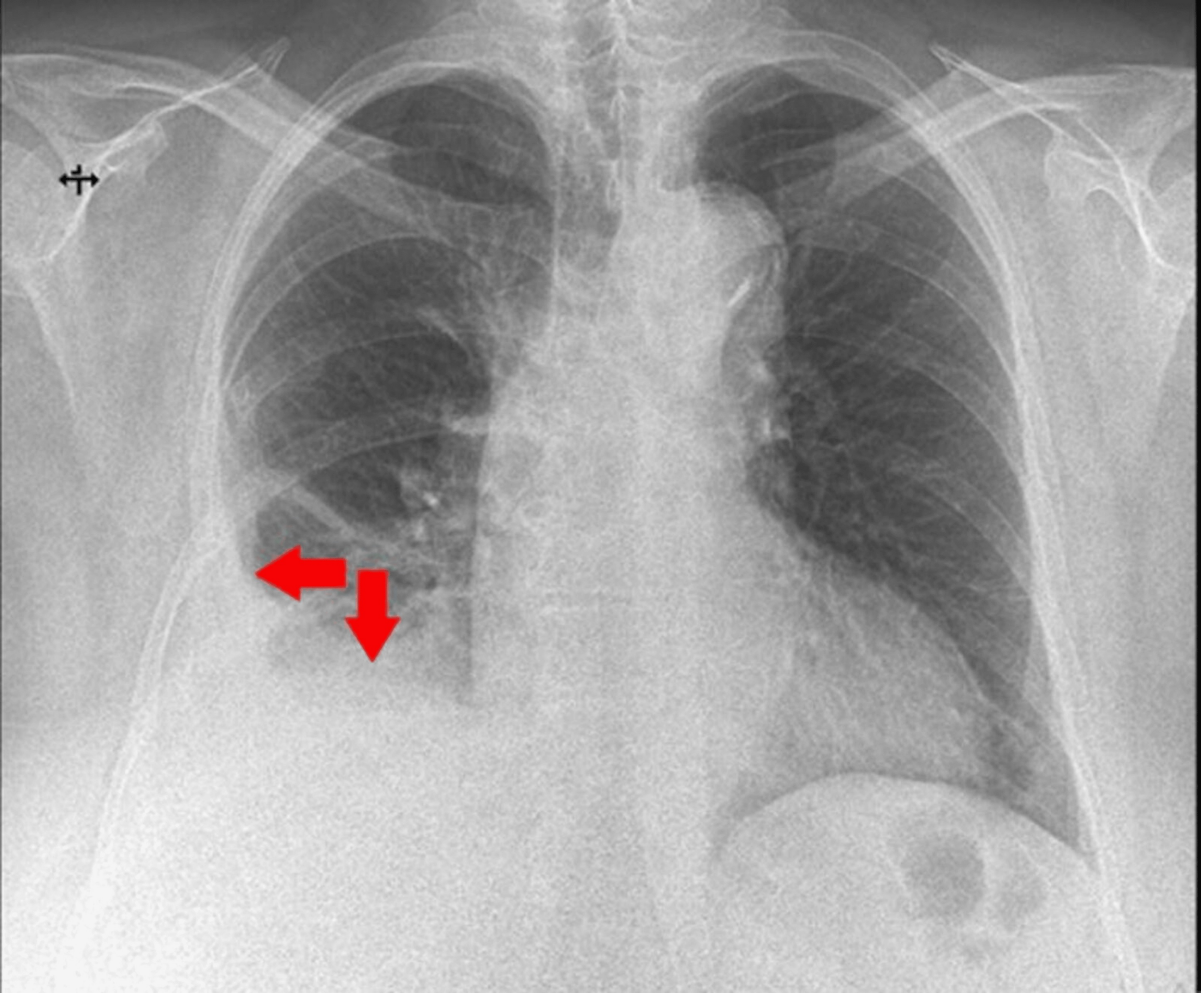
Chest X-ray (posteroanterior view), showing a small to moderate right pleural effusion
